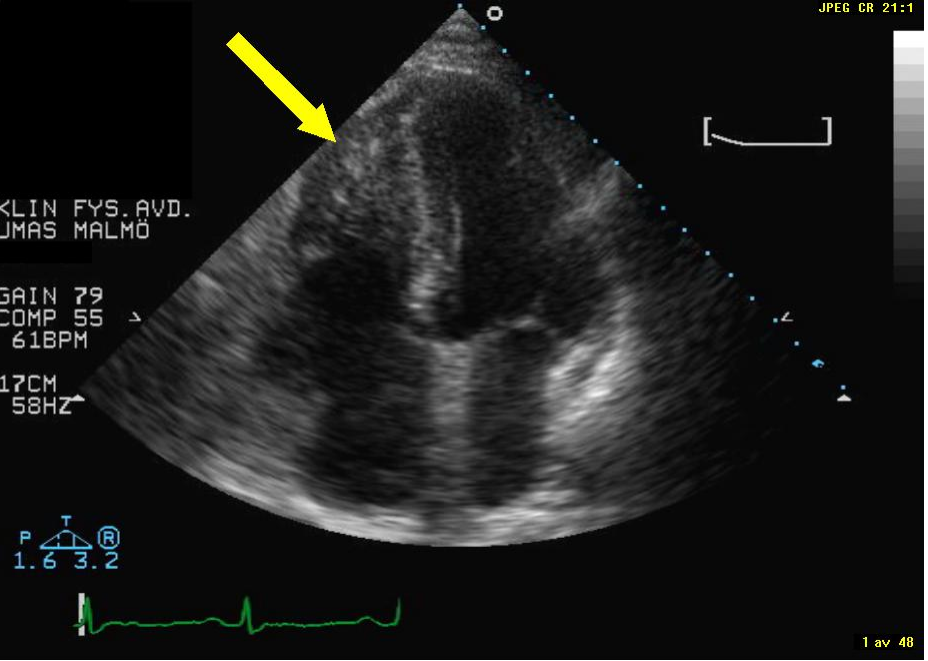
Display of echocardiography image from apical four-chamber view. The yellow arrow indicates the metastasis of the leiomyosarcoma.
